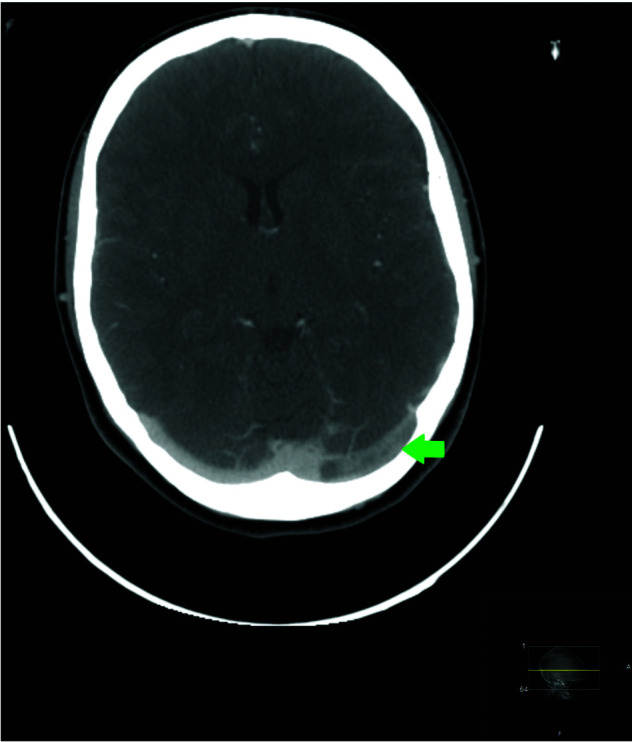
CT cerebral venography demonstrating thrombosis of the left transverse sinus. CT: computed tomography.
